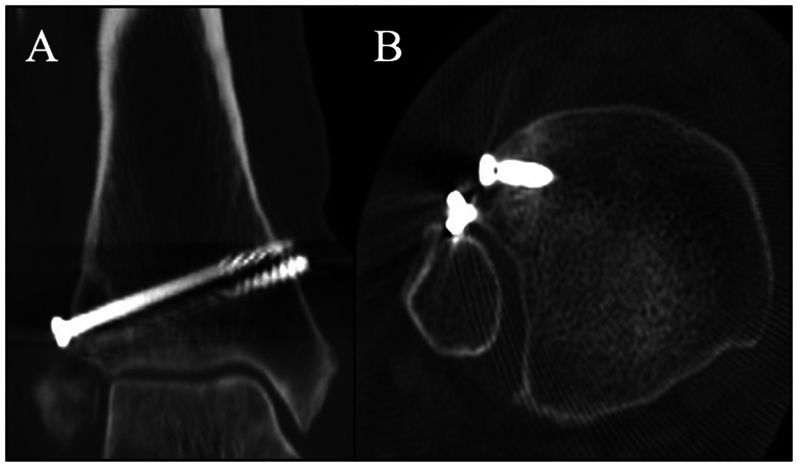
Postoperative control CT scan view: (
